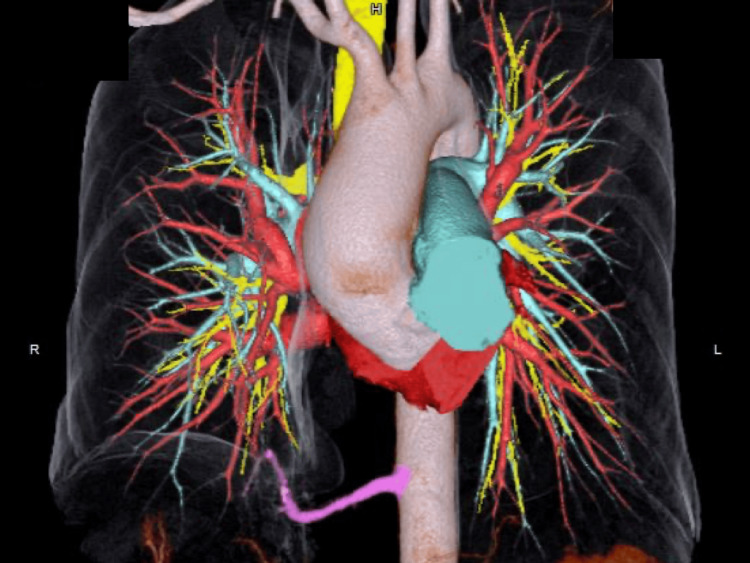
Enhanced CT revealed an abnormal artery supplying segment 10 of the right lower lobe branching from the descending aorta. The course of the bronchi was normal.
